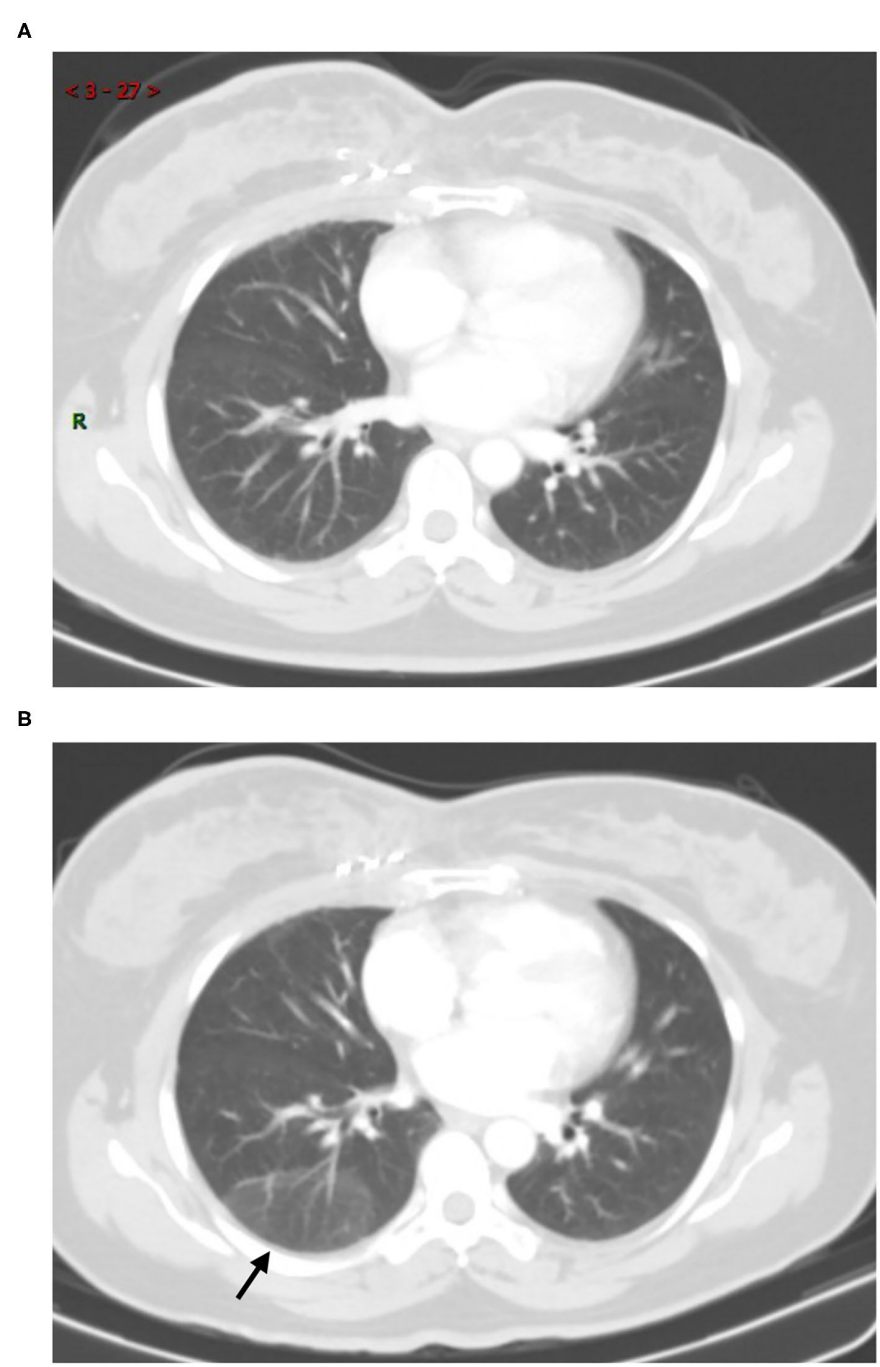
Fourty-two-year-old female with breast cancer history. Screening CT scan showed a focal peripheral rounded and ill-defined GGOs in the right lower lobe [(B), arrow]. Comparison to the prior CT scan (A), it has been newly seen.
